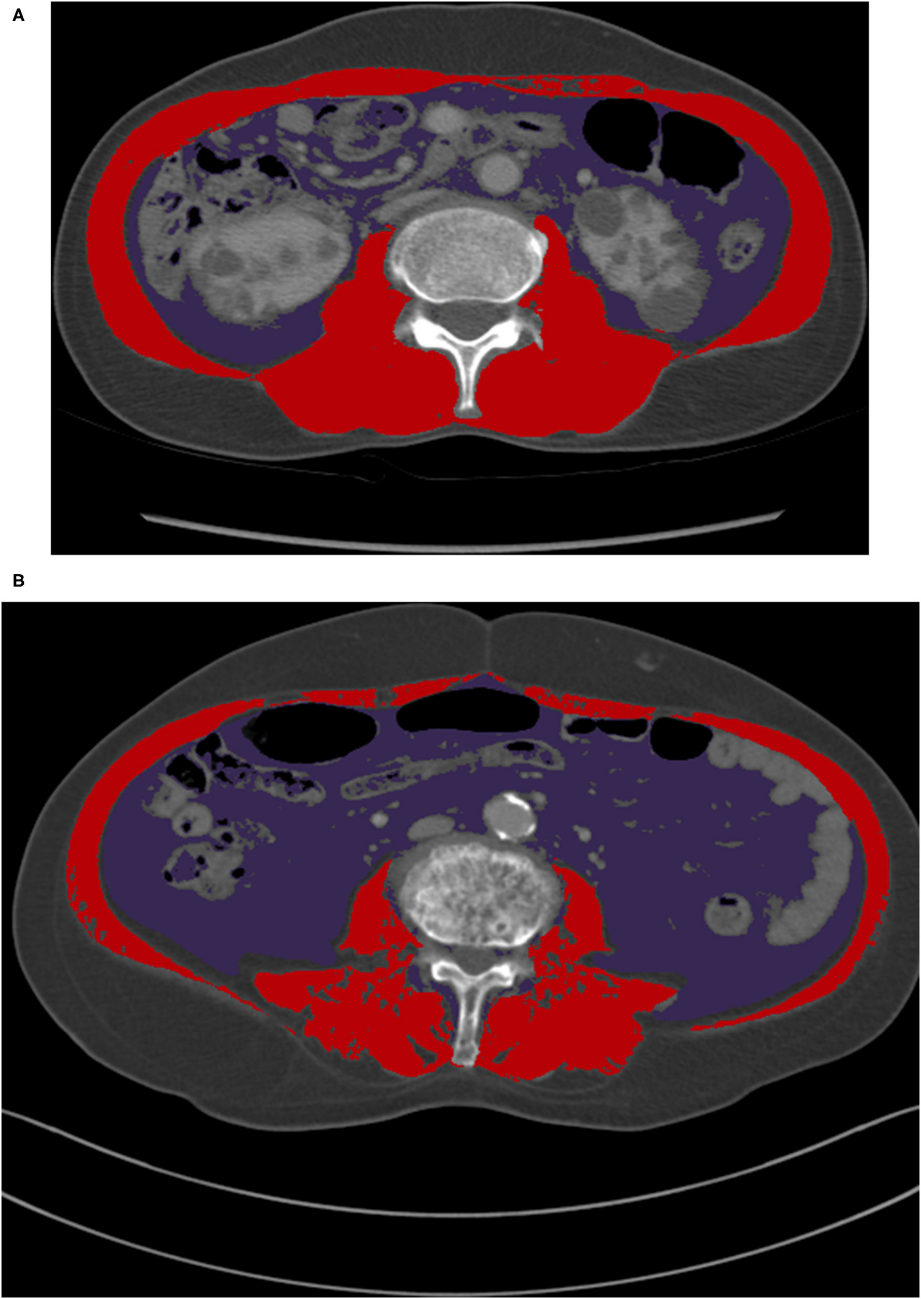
A comparison of CT images at L3 for male patients with normal BMI categories. (A) A patient who had skeletal muscle depletion only (SMI of 38.3 cm^2^/m^2^ and VFA of 61.5 cm^2^), (B) A patient who had visceral fat obesity and skeletal muscle depletion (SMI of 35.9 cm^2^/m^2^ and VFA of 100.1 cm^2^). BMI, body mass index; CT, computed tomography; L3, the third lumbar vertebra; SMI, skeletal muscle index; VFA, visceral fat area.
